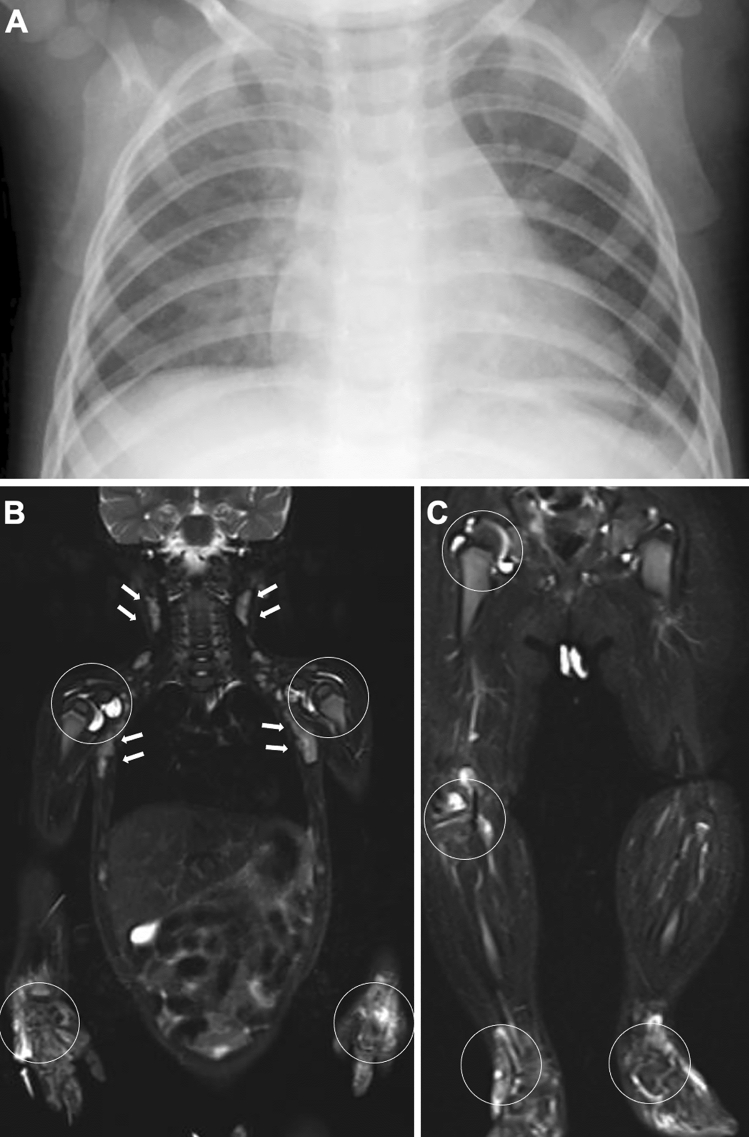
Systemic JIA. A–C. A 10-month-old boy with fever, rash, and multiple joint swelling. Frontal chest radiograph showed cardiac enlargement and mild pulmonary edema (A). Whole body STIR images (B, C) demonstrated bilateral symmetrical polyarthritis (increased joint fluid and edema of the carpal region) (circles, B, C) as well as cervicoaxillary lymphadenopathy (arrows, B)
